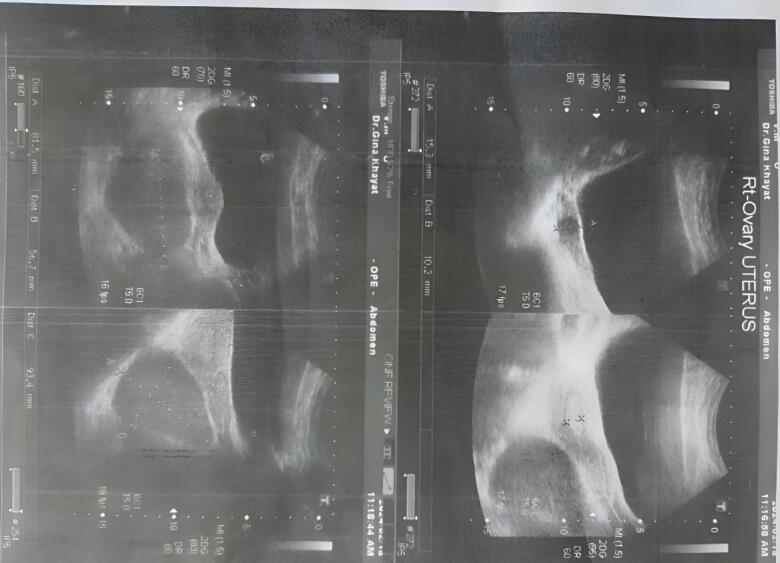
Abdominal ultrasound showing a large cyst behind the cervix.
